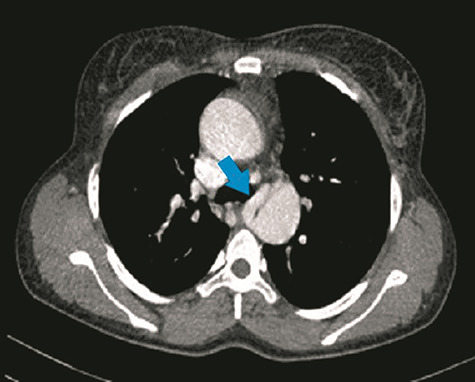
CT chest axial view shows first collateral artery (blue arrow) arising from the right margin of the descending aorta at the level of the carina.
